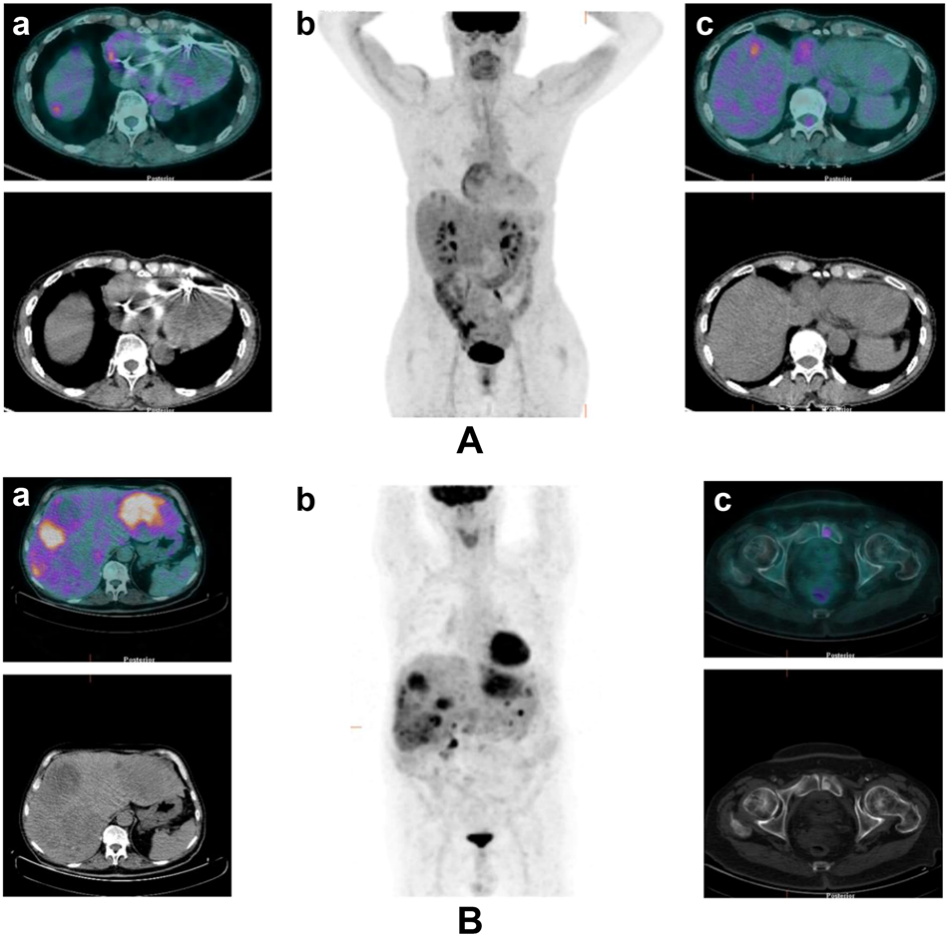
Two cases of patients with positive [^18^F]FDG PET /CT. A A 78-year-old female patient with newly diagnosed ileal NET G1 with liver metastases. (a, c) Axial FDG- PET/CT and (b) maximum intensity projection (MIP) images showing pathological uptake in two liver lesions. B A 73-year-old-male patient affected by pancreatic NET G1 with liver, lymph node, and bone metastases. (a) Axial [^18^F]FDG PET/CT and (b) maximum intensity projection (MIP) images showing pathological uptake in several liver lesions and in small bone lesion (c). Abbreviation: [^18^F]FDG PET/CT fluorodeoxyglucose positron emission tomography/computed tomography
